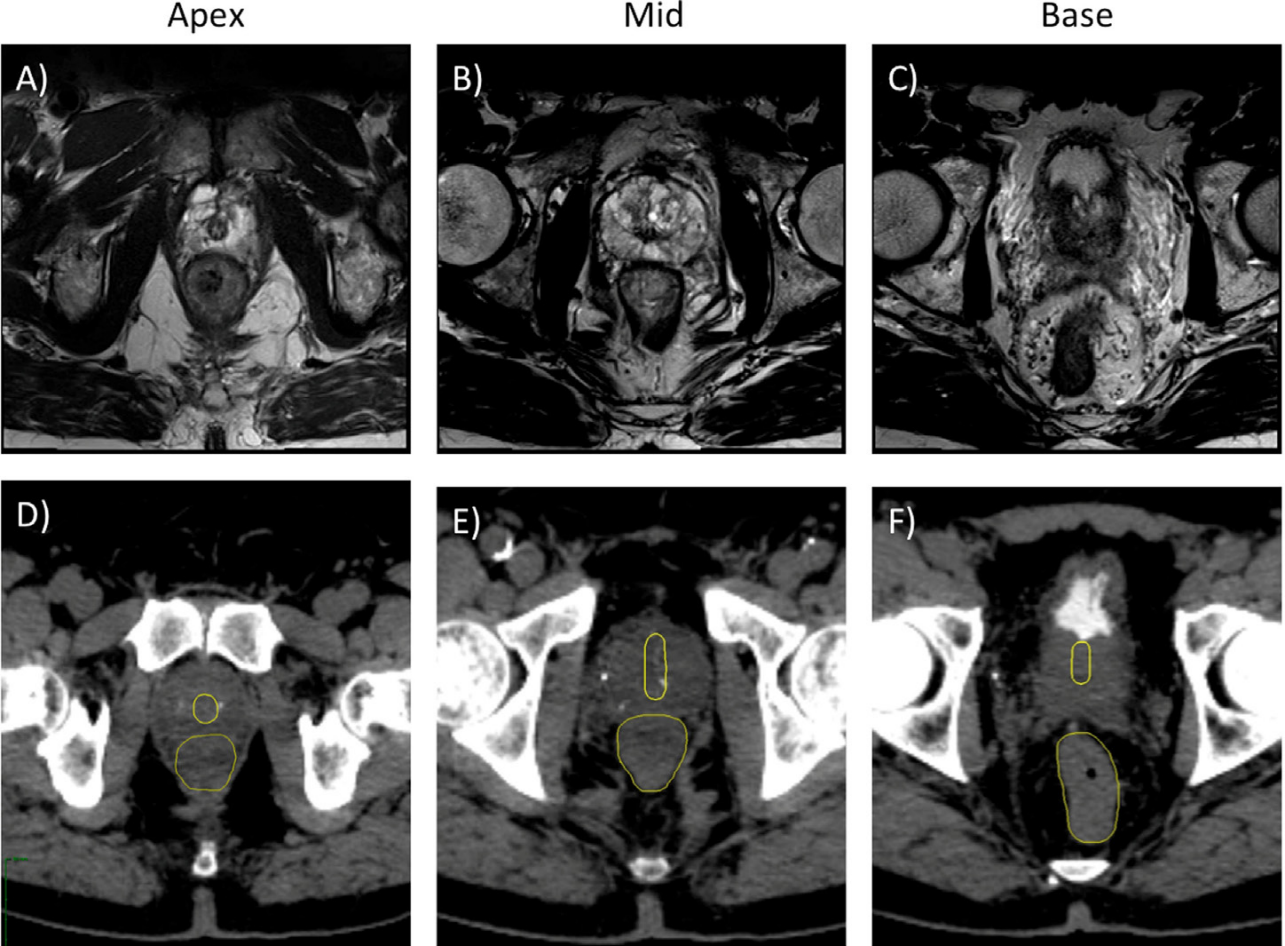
Treatment planning axial T2-weighted MR (A–C) and axial CT (D–F) images demonstrating the prostatic urethra and rectum. (A,D) Axial plane, 0.5 cm cranial to apex. (B,E) Axial plane, mid-prostate level. (C,F) Axial plane, 1 cm caudal to base.
